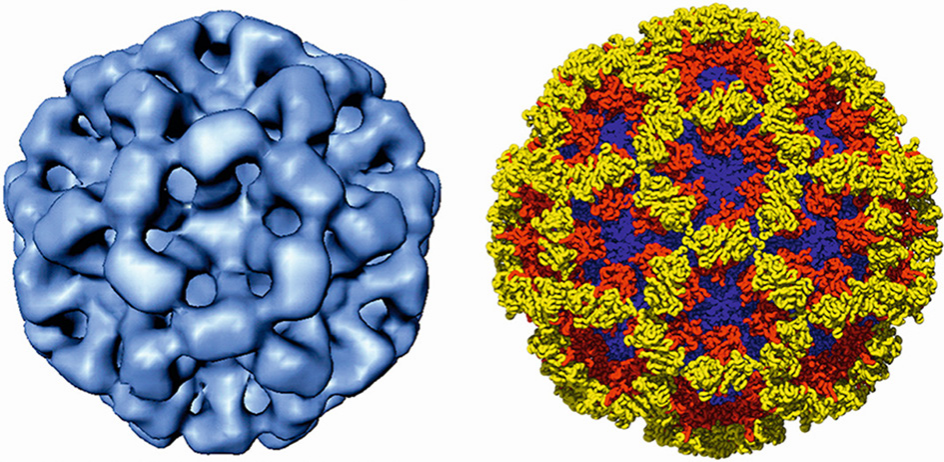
The structure of the calicivirus capsid exemplified by a cryo-image reconstruction of recombinant Norwalk virus-like particles (left). X-ray structure of the Norwalk virus capsid (right) with the shell, protruding 1 and protruding 2 domains shown in blue, red and yellow, respectively. (Courtesy of B. V. Prasad.)
